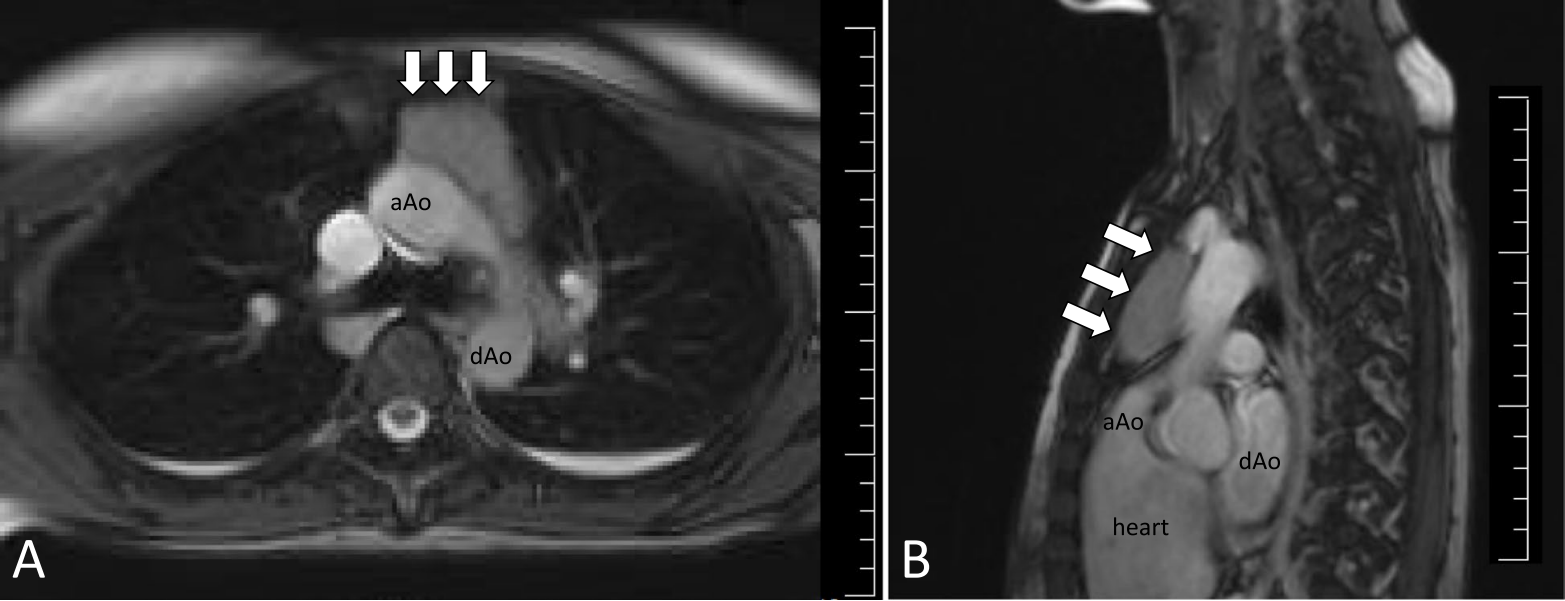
T2-weighted magnetic resonance imaging. A Axial view. B Sagittal view. The images show a soft tumor mass (marked by white arrows, measuring 46 × 46 × 22 mm) in the anterior mediastinum. aAo, ascending aorta; dAo, descending aorta
